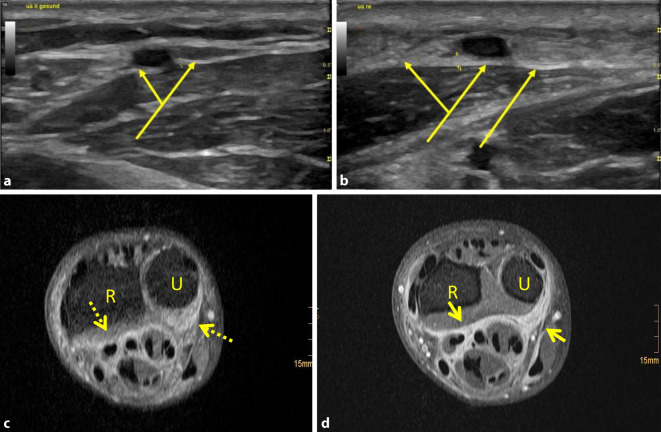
In der hochauflösenden Sonographie mit einem 18 MHz Hockey-Stick des rechten Unterarms (Abb. 2b) zeigte sich ein typischer Befund verdickter Faszien im Bereich der beschriebenen Verhärtung am rechten Unterarm. Zum Vergleich zeigt Abb. 2a den unauffälligen linken Unterarm.
